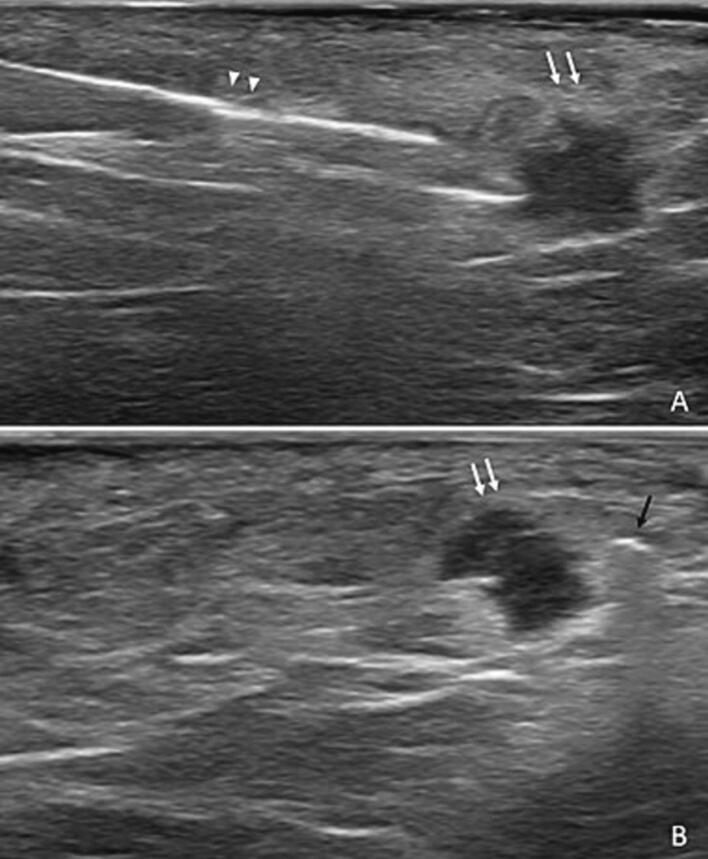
Magnetic seed implantation under ultrasound guidance. After a local disinfection and anesthesia, the magnetic seed (black arrow) was implanted next to the tumoral lesion (white arrows) using a 14G sterile needle (arrowhead), under ultrasound guidance. Neither immediate nor delayed complications occurred.
